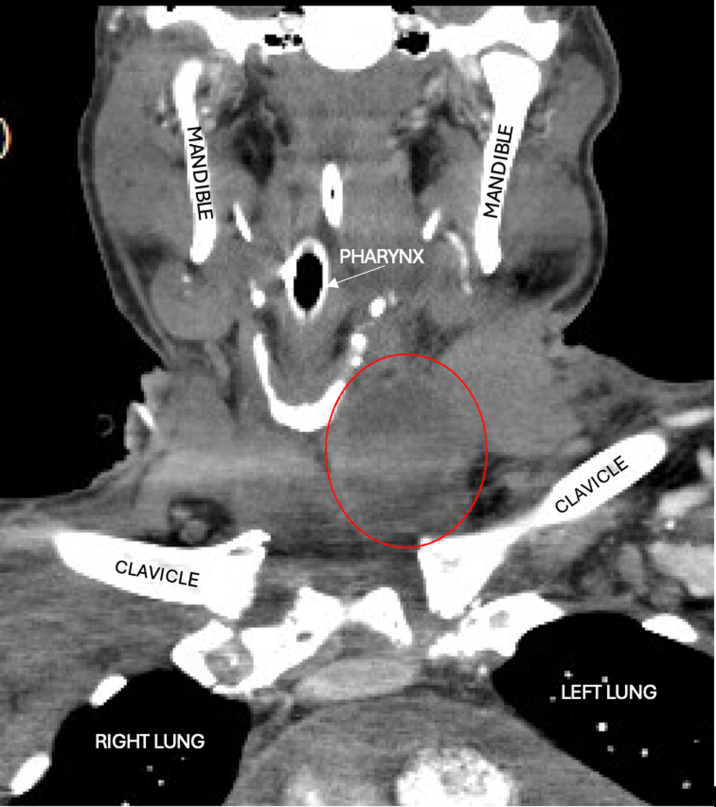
Computed Tomography of the Left Neck With Contrast Computed tomography coronal view of the chest/neck with contrast showing a rim-enhancing fluid collection (red circle) in the left neck.
